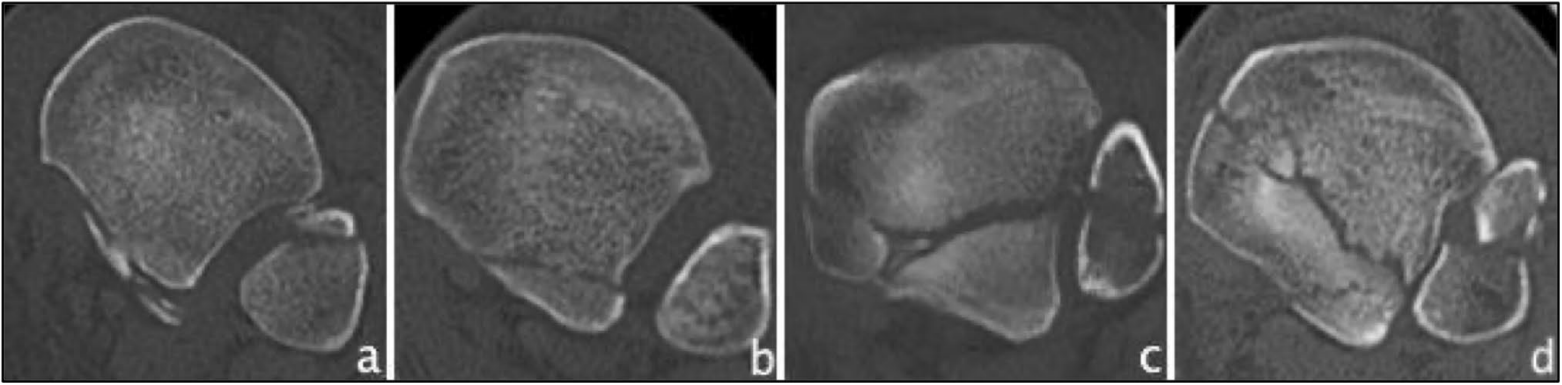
Overview of the Mason classification based on CT images (axial views). a Mason type 1 b Mason type 2A c Mason type 2B d Mason type 3
